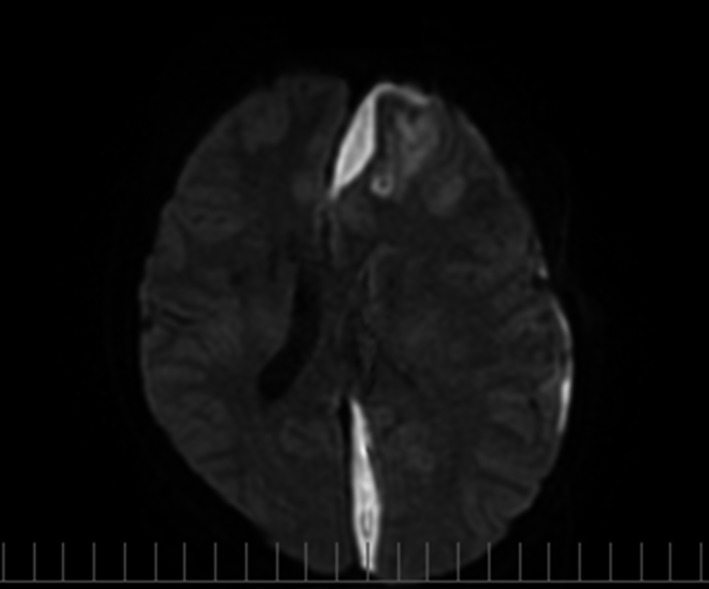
Follow‐up magnetic resonance imaging studies performed on day 7 (4 days after burr hole craniotomy). Diffusion‐weighted image shows enlargement of the subdural abscess without improvement of the midline shift even after burr hole craniotomy.
